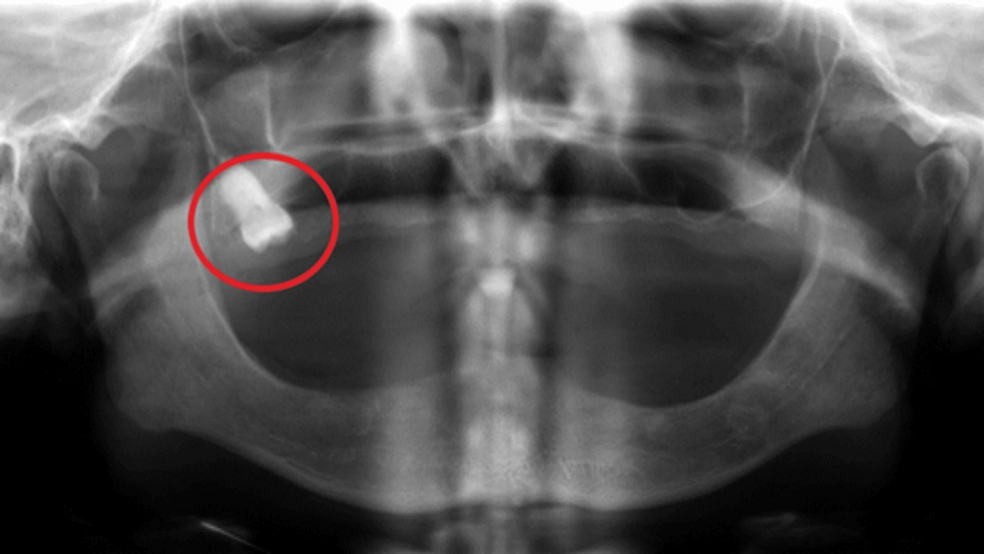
Impacted tooth as seen on a panoramic radiograph
